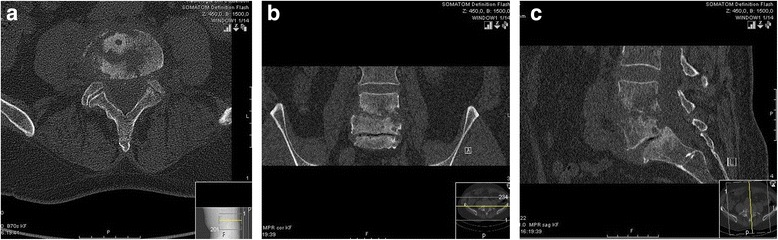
a, b and c Preoperative computed tomography scan of the lumbar spine on 6 Jan 2015 (axial (*left*), coronary (*middle*) and sagittal (*right*) reconstructions): significant bone osteolysis and erosion of base plate L4 and upper plate L5
